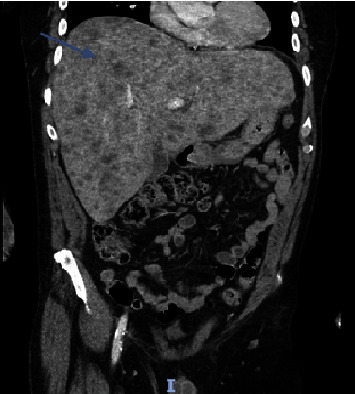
Coronal section obtained from computed tomography of the abdomen and pelvis shows numerous hypovascular liver masses measuring up to 4.8 cm (blue arrow).
